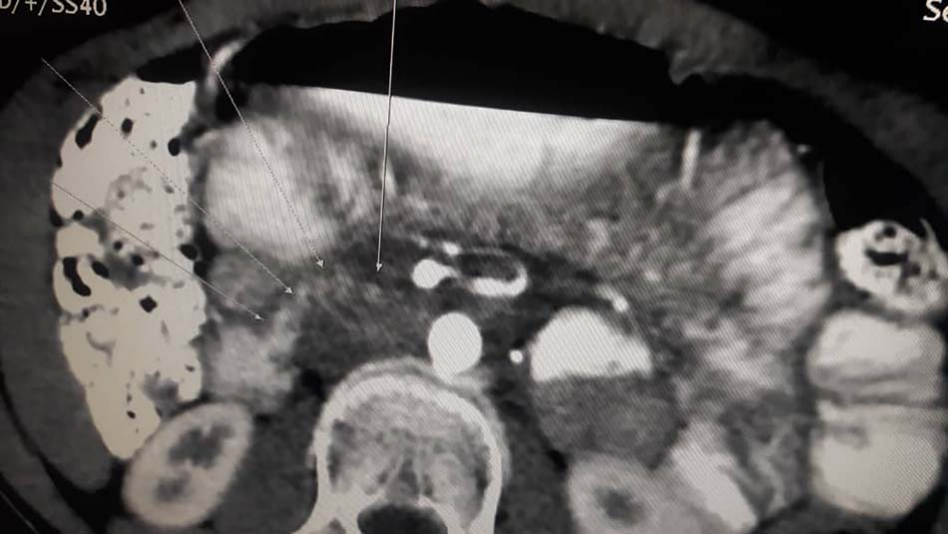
Contrast enhanced CT scan abdomen and pelvis (sagittal view) (axial view) showing duodenal compression between the aorta and superior mesenteric artery.
